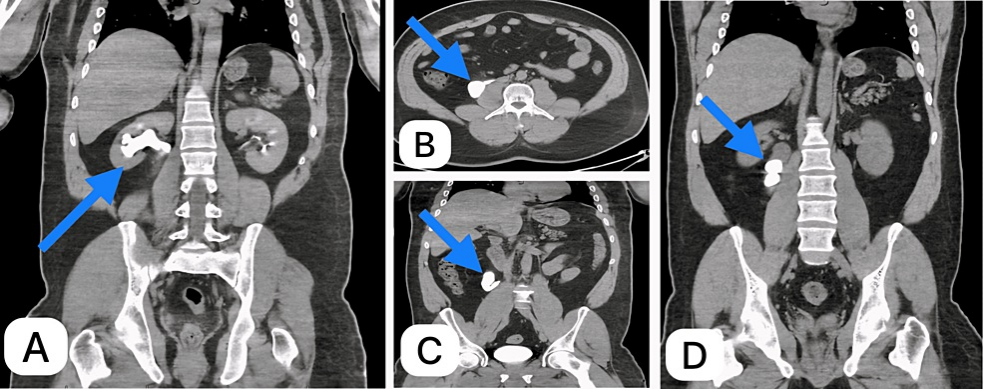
Computed tomography (CT) scan Blue arrows show (A) a coronal image of CT KUB with contrast showing a dilated ureter and renal pelvis, (B) an axial image of CT with contrast delayed film showing a ureter behind the IVC, (C) a coronal image of CT KUB with contrast delayed film showing a dilated upper ureter and hooked-shape ureter, and (D) coronal plain CT KUB showing right upper ureteric stones with a dilated upper ureter KUB, kidney, ureter, and bladder; IVC, inferior vena cava
